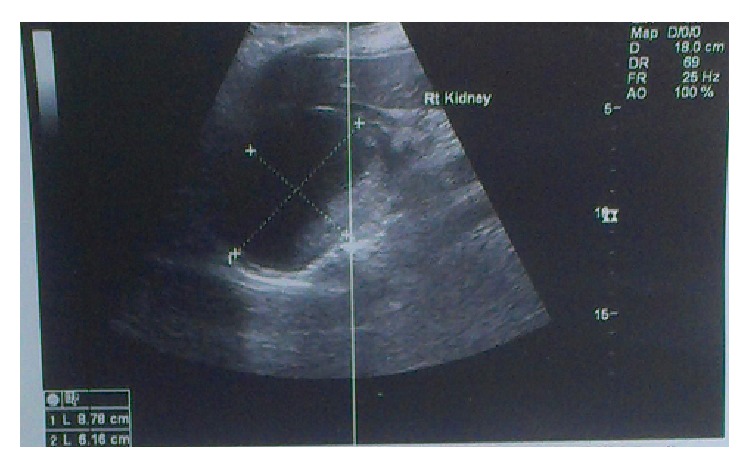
Ultrasound of the abdomen. Tumor between right kidney and liver, with mixed echogenicity, low vascularity, and dimensions 9 × 7.5 cm.
